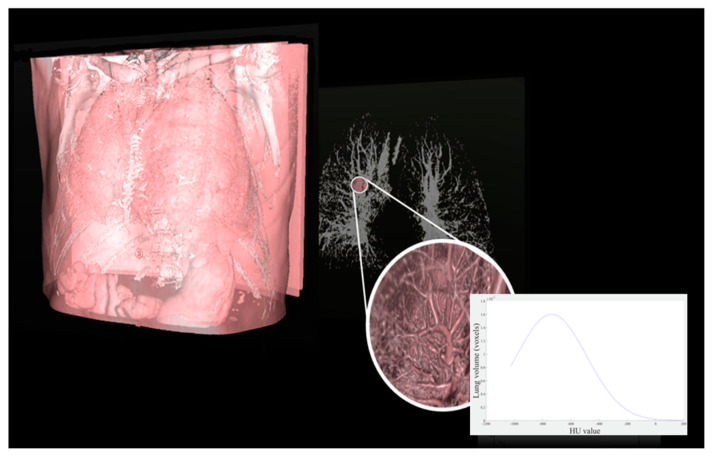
Representative high-resolution computed tomography (HRCT) study and extraction of lung histogram to obtain quantitative parameters.
